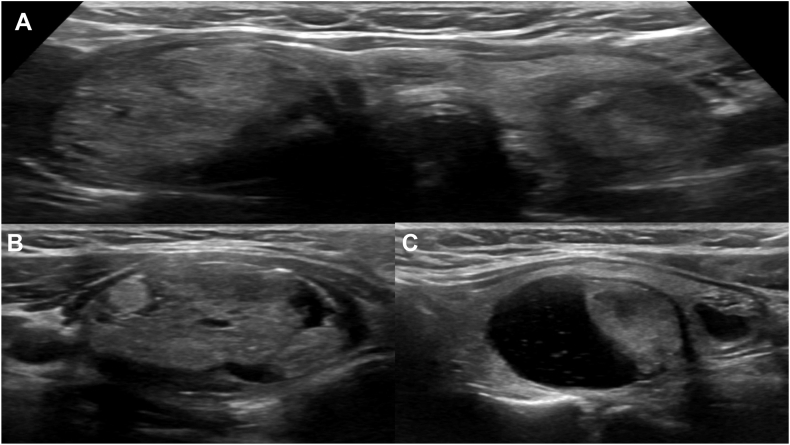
Preoperative thyroid ultrasound. *A*, The transverse view of the thyroid ultrasound shows bilateral thyroid nodules. *B*, *Right* thyroid nodule (32 mm)—isoechoic, mixed cystic and solid, wider than tall, with a smooth margin and punctate echogenic foci (TIRADS 4). *C*, *Left* thyroid nodule (27 mm)—mixed cystic and solid, hypoechoic, taller than wide, with a smooth margin, and without echogenic foci (TIRADS 5). *TIRADS* = Thyroid Imaging Reporting and Data System.
